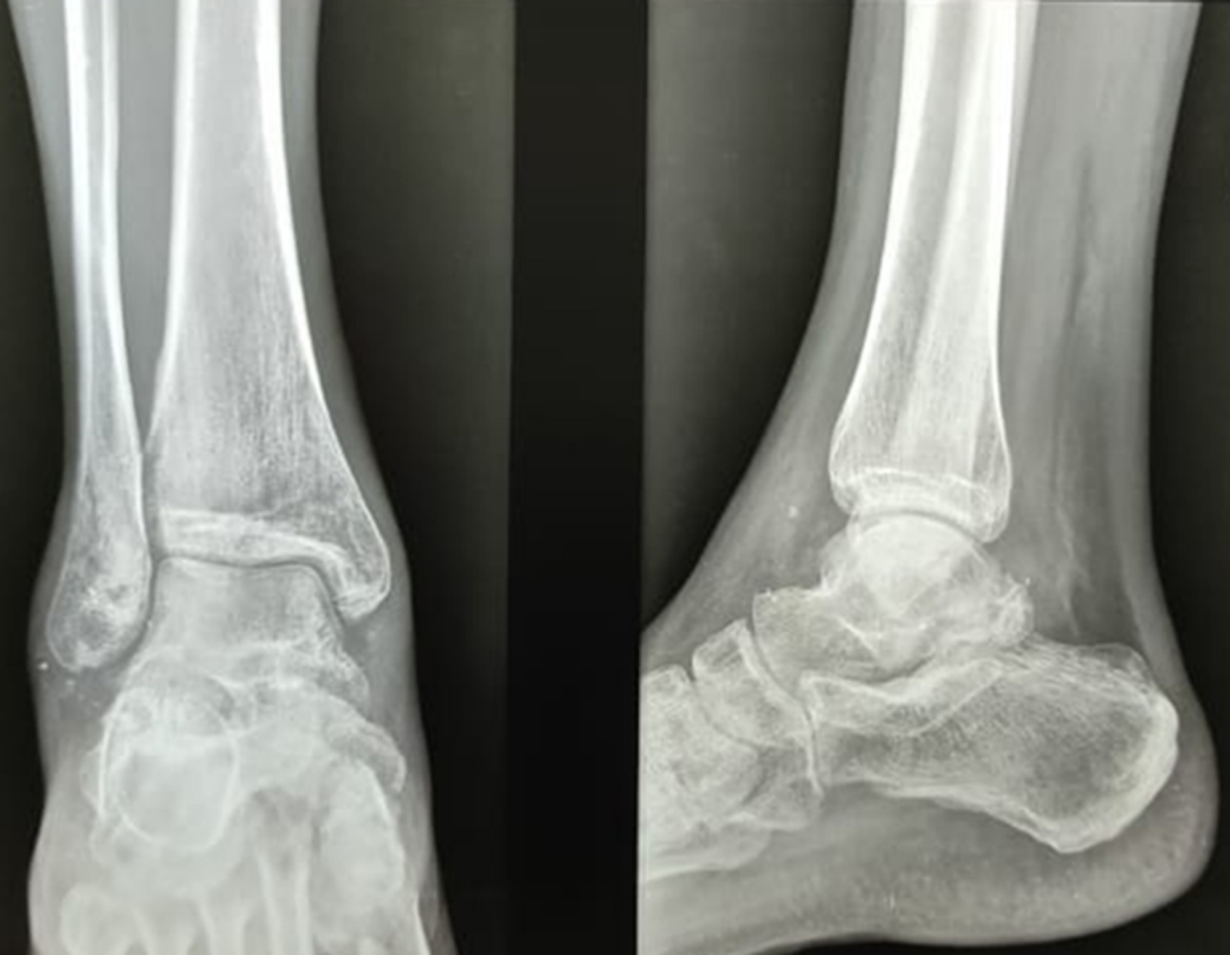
Plain radiographs of right ankle (anteroposterior and lateral views) at one-year post-injury. Hawkins sign is positive, suggestive of good vascularity of the talus.
